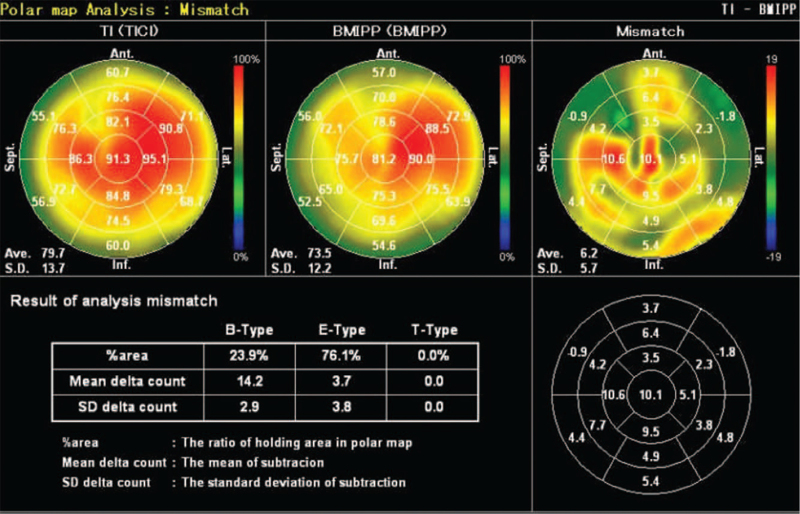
^123^I-BMIPP/^201^TlCl scintigraphy images reveal mismatch in ^123^I-BMIPP and ^201^TlCl uptake.
